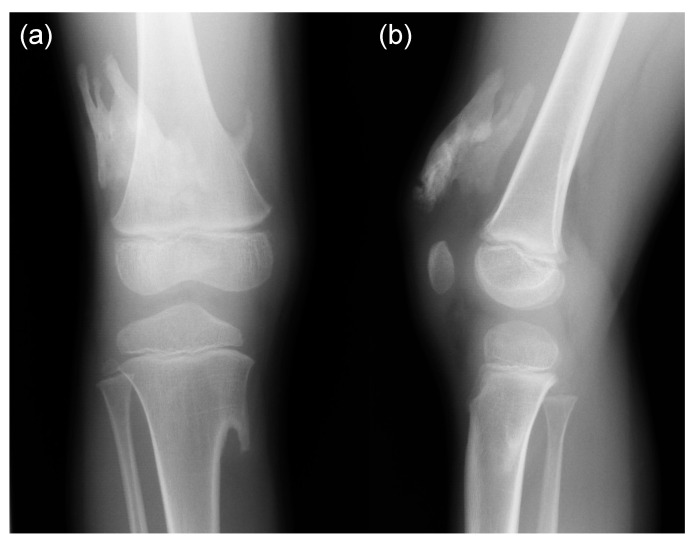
Anteroposterior (a) and lateral (b) knee radiographs of the FOP boy at the age of 5 years showing heterotopic ossification at the distal thigh and osteochondroma-like lesions of the distal femur and proximal tibia.
